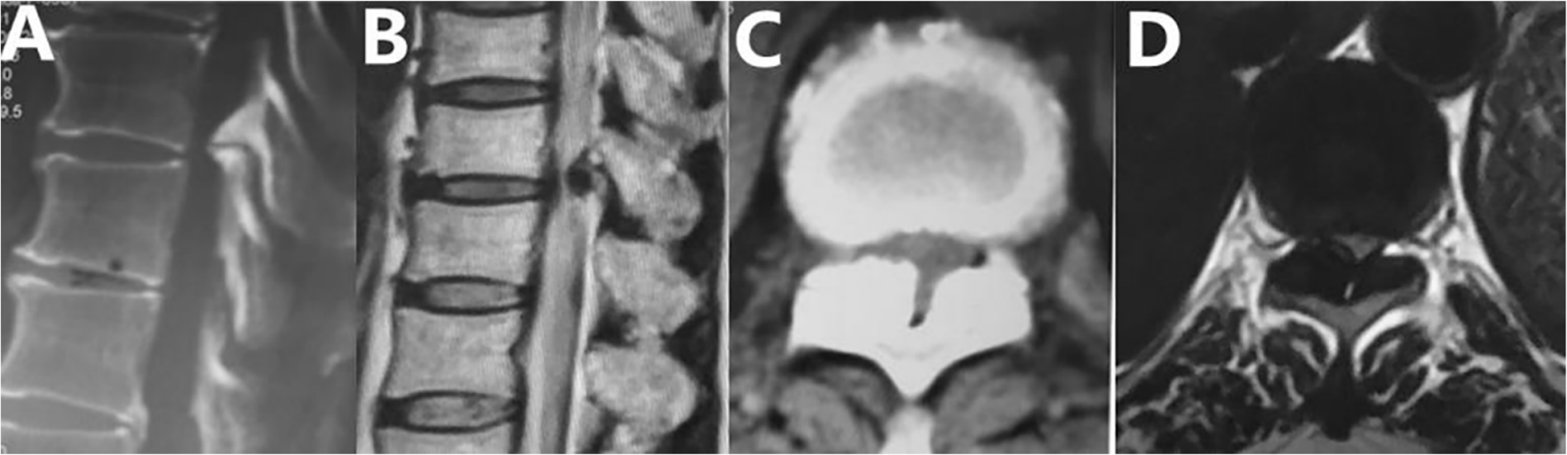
Postoperative CT and MRI. Satisfactory decompression was performed
